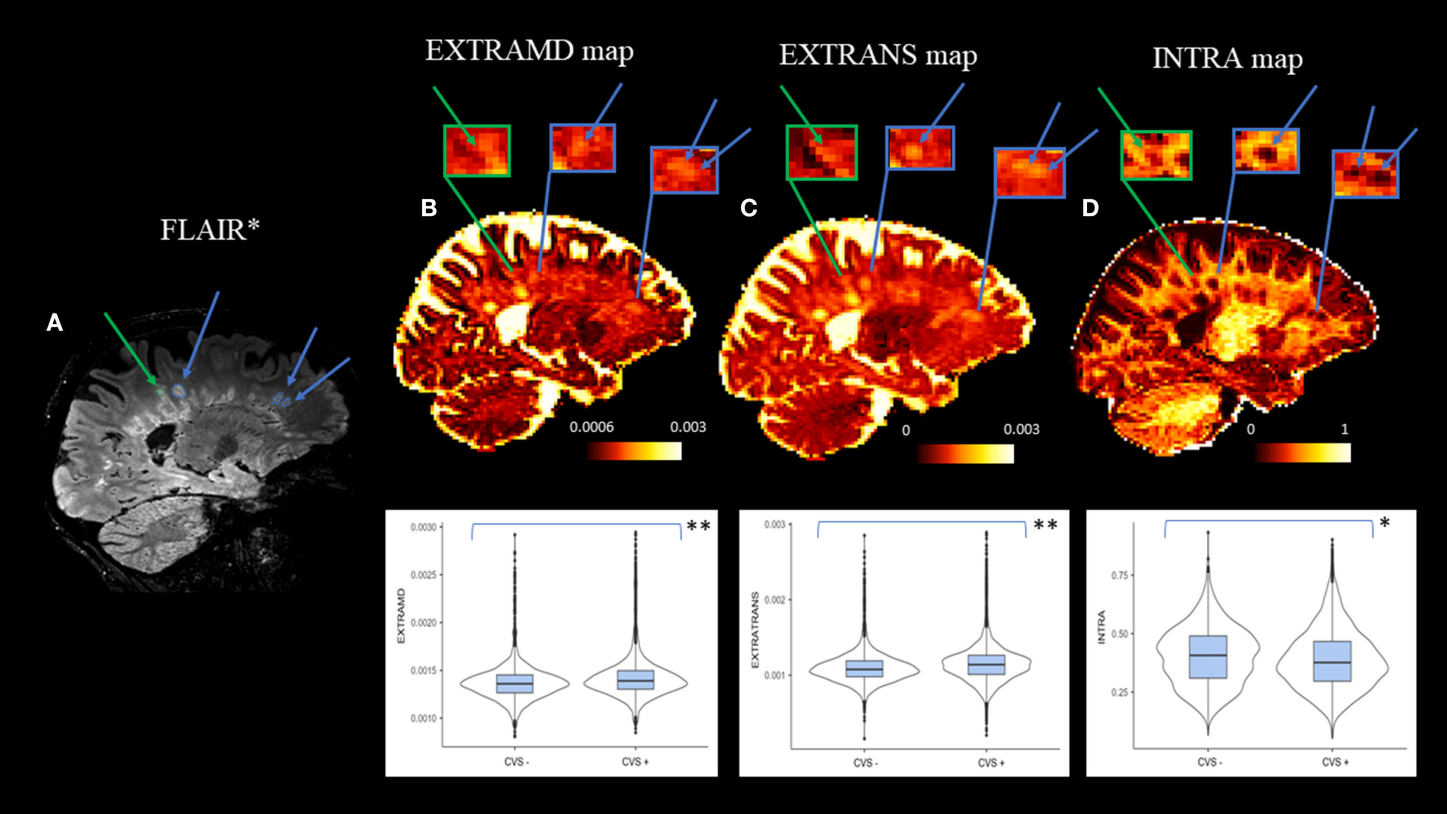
Selected sagittal FLAIR* (A) and SMT-derived EXTRAMD (B), EXTRATRANS (C) and INTRA (D) maps of a 62-year-old patient diagnosed with multiple sclerosis. At the top: the color bar expresses each SMT-metric: adimensional unit for INTRA (intraneurite signal fraction, corresponding to fiber disruption), mm^2^/sec for EXTRAMD (extraneurite mean diffusivity, corresponding to inflammation severity), and EXTRATRANS (extraneurite transverse diffusivity, corresponding to the degree of demyelination) measures. Arrows and zoomed-in boxes indicate the presence of representative CVS+ lesions (blu arrows) and CVS− lesion (green arrows) to highlight the differences in SMT metrics among them. At the bottom: violin plots showed higher inflammation, demyelination, and fiber disruption in CVS+ lesions compared to CVS− lesions (*p* = 0.001; p* = 0.02). CVS, Central Vein Sign.
